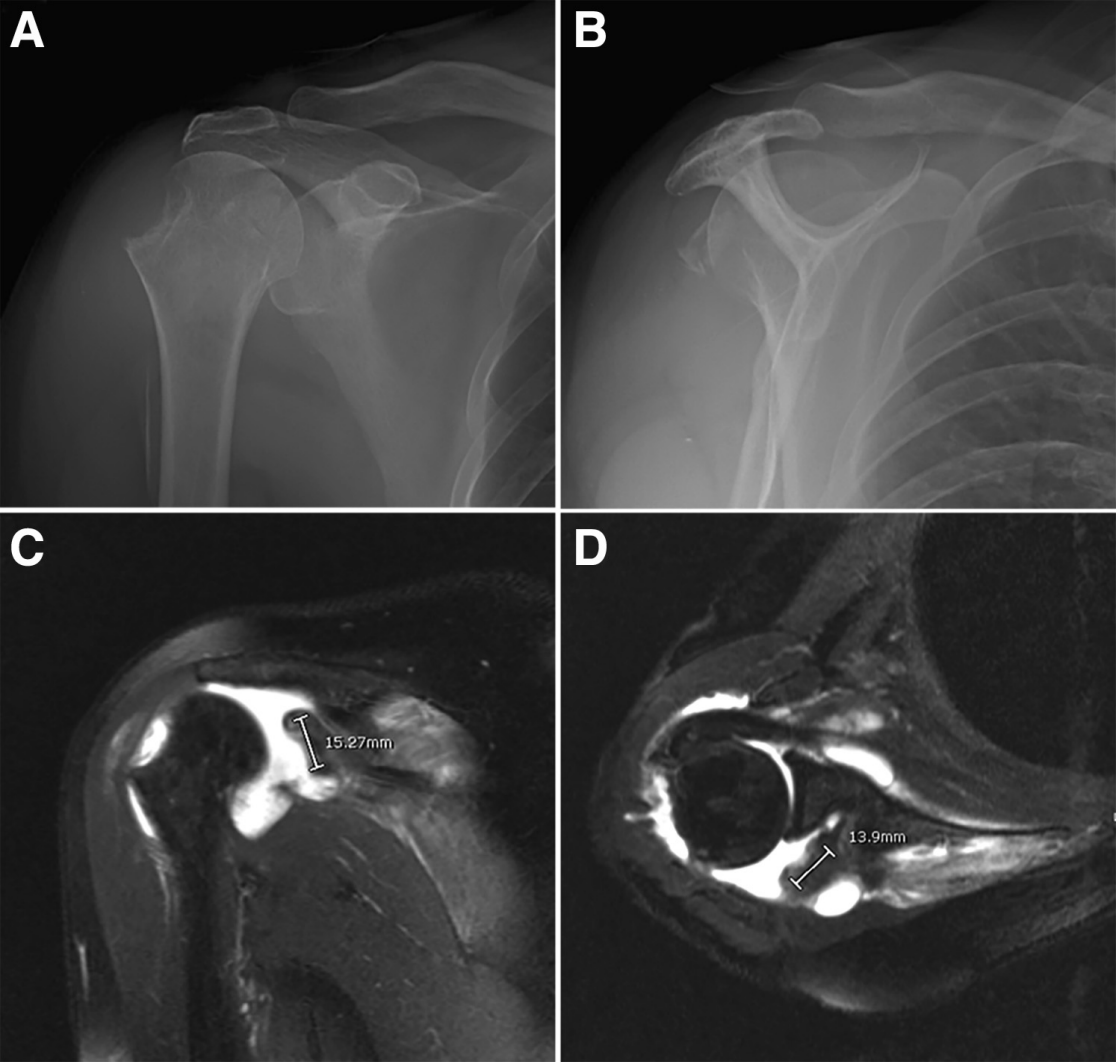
A patient presented with chronic severe retraction of the greater tuberosity avulsion fractured right shoulder. (A) Radiograph, anteroposterior view showed medial displaced greater tuberosity fracture. (B) Radiograph, lateral view showing posterior displaced greater tuberosity fracture. (C, D) Magnetic resonance imaging (1.5 Tesla). (C) Oblique coronal T2-weighted with fat saturation showed medial displacement of avulsion fragment. (D) Axial T2-weighted with fat saturation showed posterior displacement of avulsion fragment.
